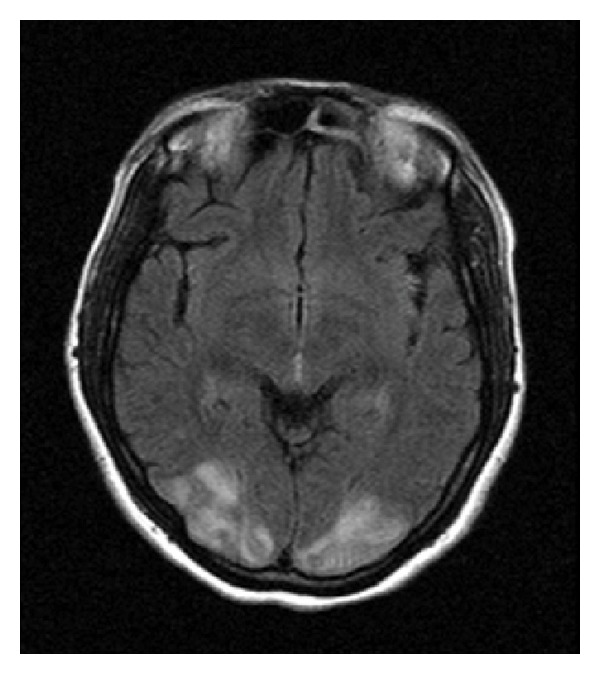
FLAIR axial images: hyperintense signal involving the white matter typically in occipital regions.
